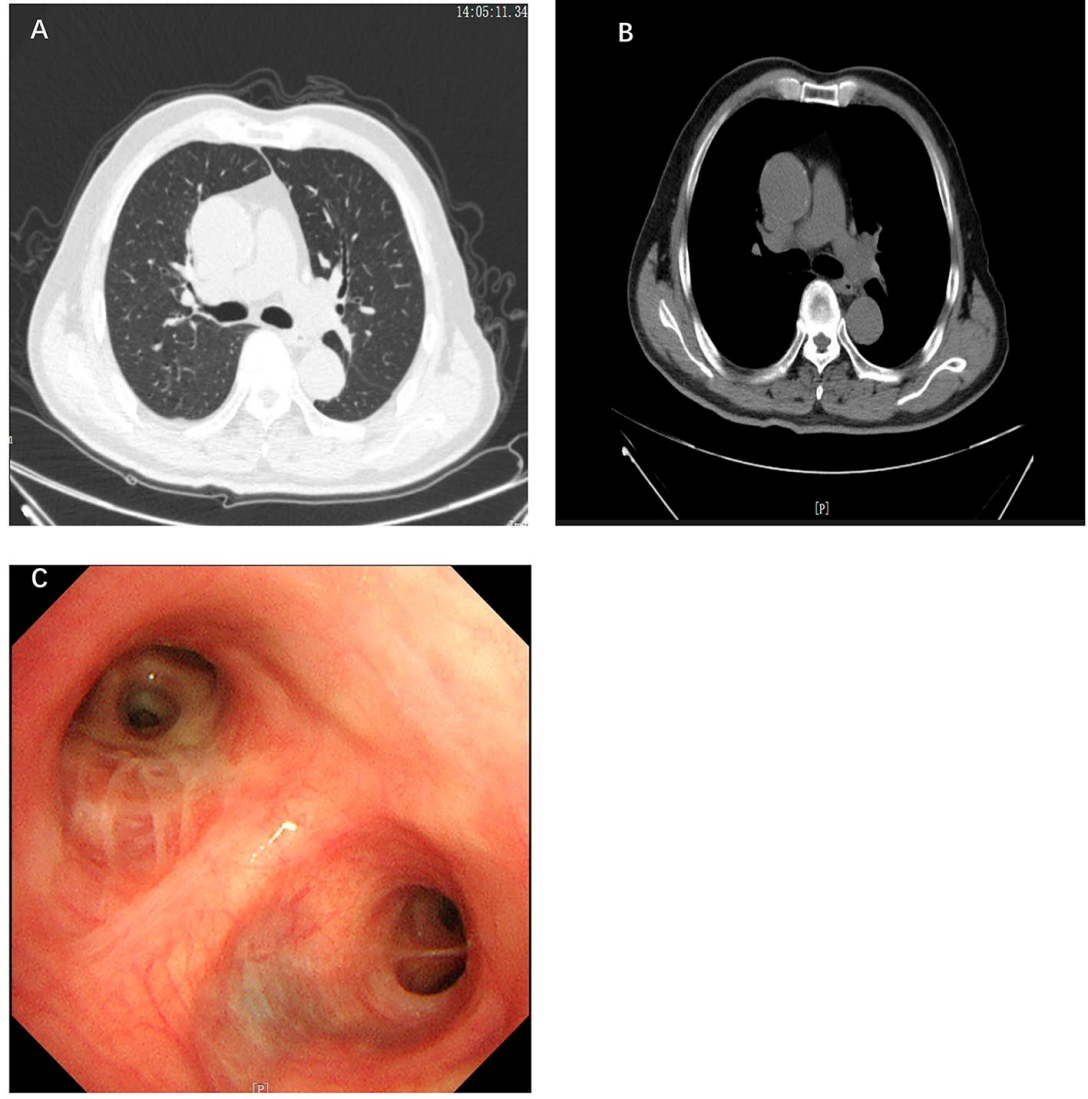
(A-B) Repeat chest computed tomography scan on 16 October 2023. A: Lung window; B: Mediastinum windows; C: Tracheoscopy was reviewed on 11 October 2023
